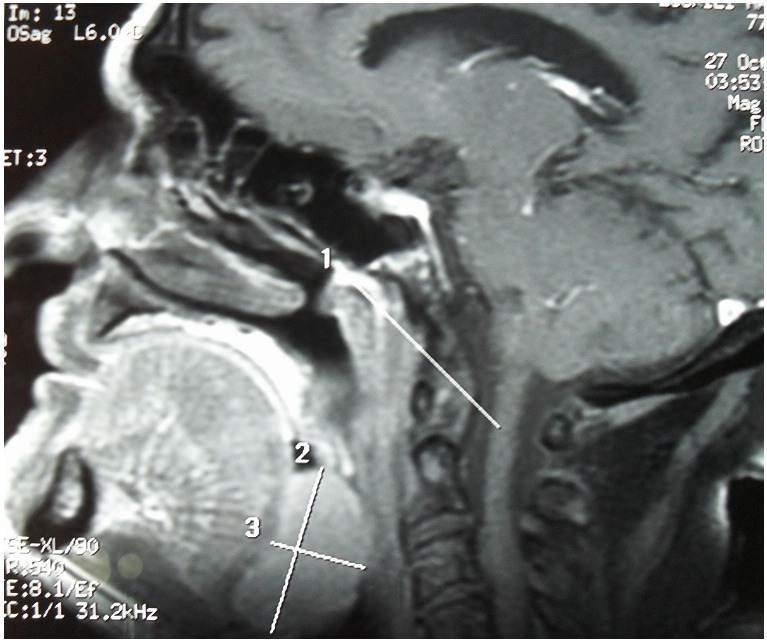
MRI showed a raised mass in the base of the tongue which filled and reduced the light of the oropharynx and the left vallecula
